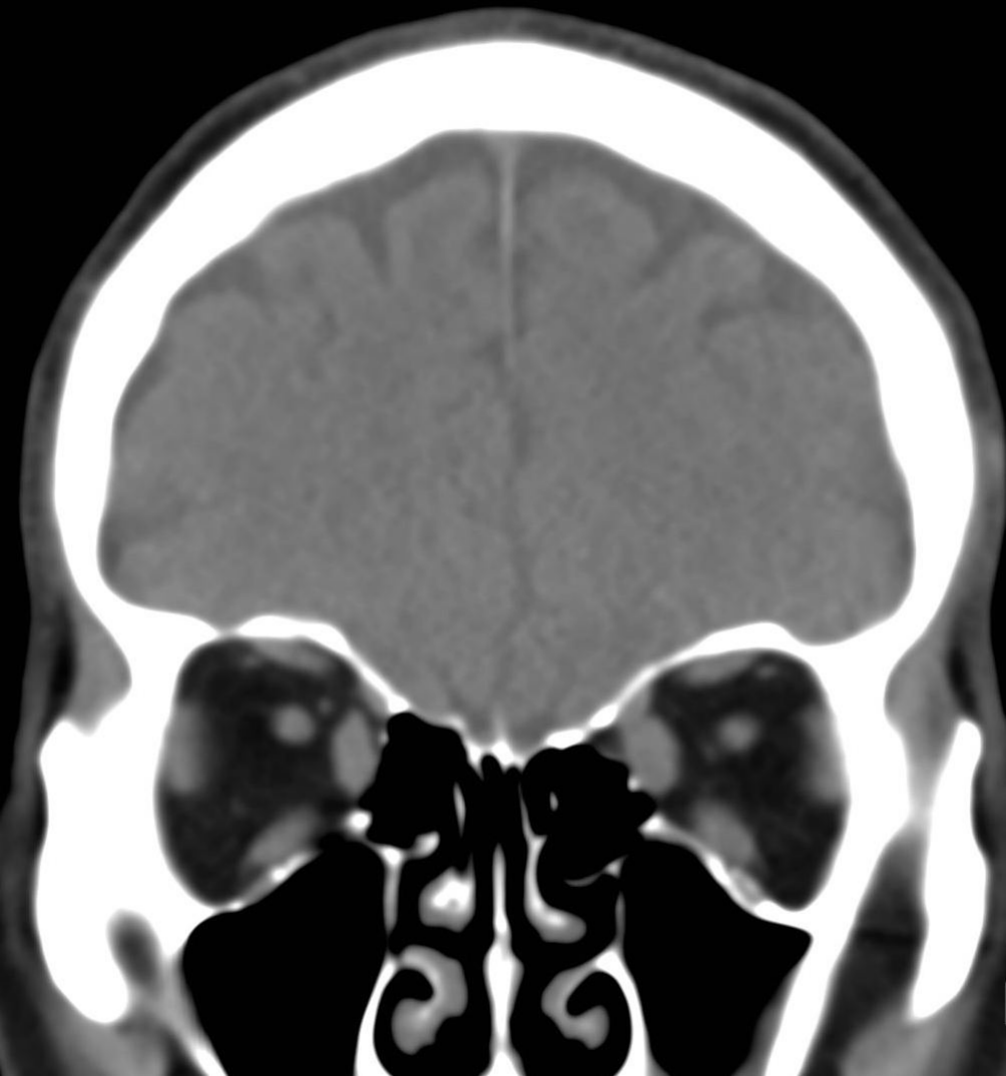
Coronal non-enhanced CT of the head demonstrates near complete resolution of bilateral extraocular muscle enlargement after completion of treatment for primary lung squamous cell carcinoma.
